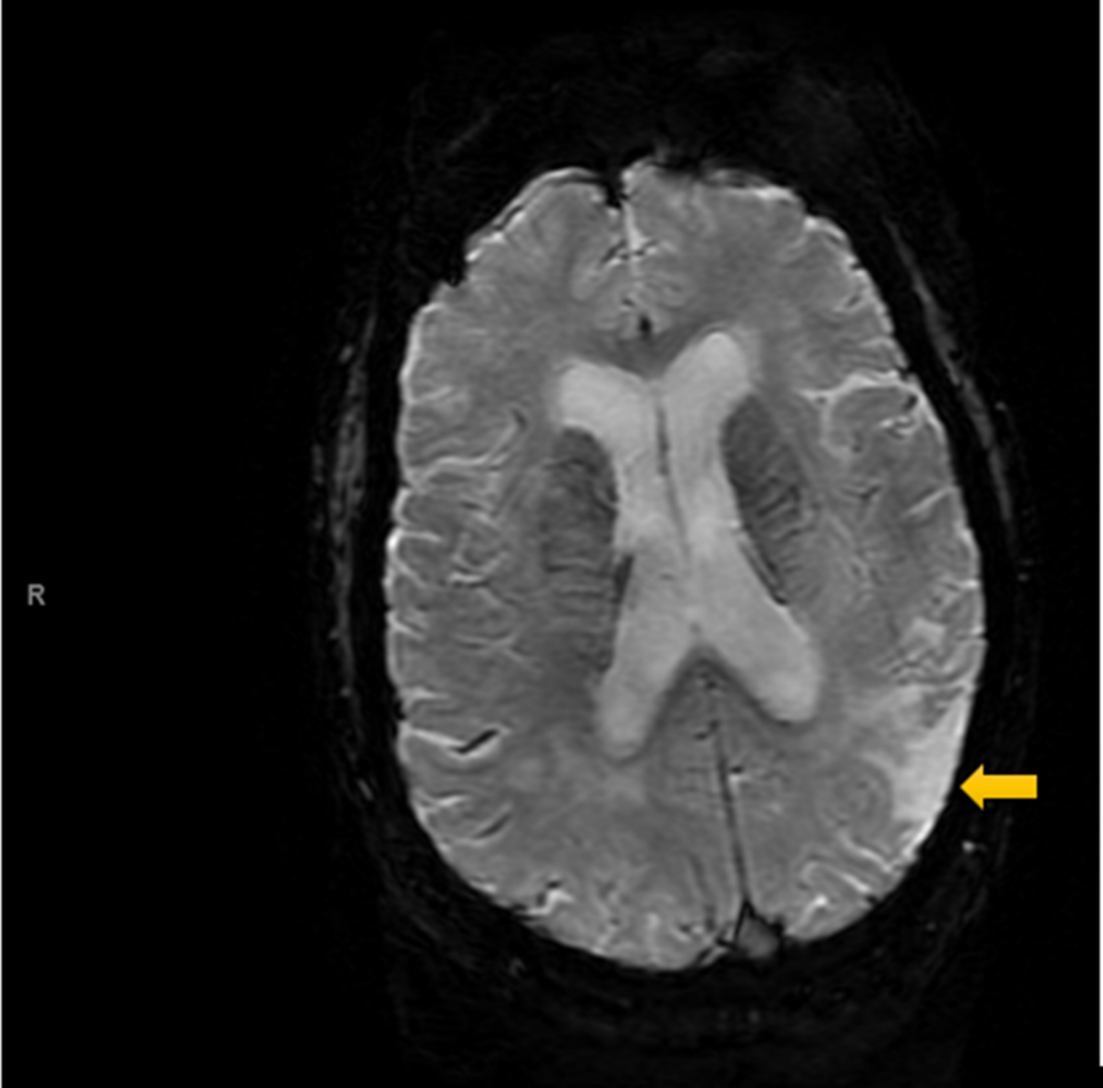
Susceptibility-weighted MRI Scan of the Head Non-contrast susceptibility-weighted MRI showing an old infarct in the left parietal-temporal-occipital lobe.
